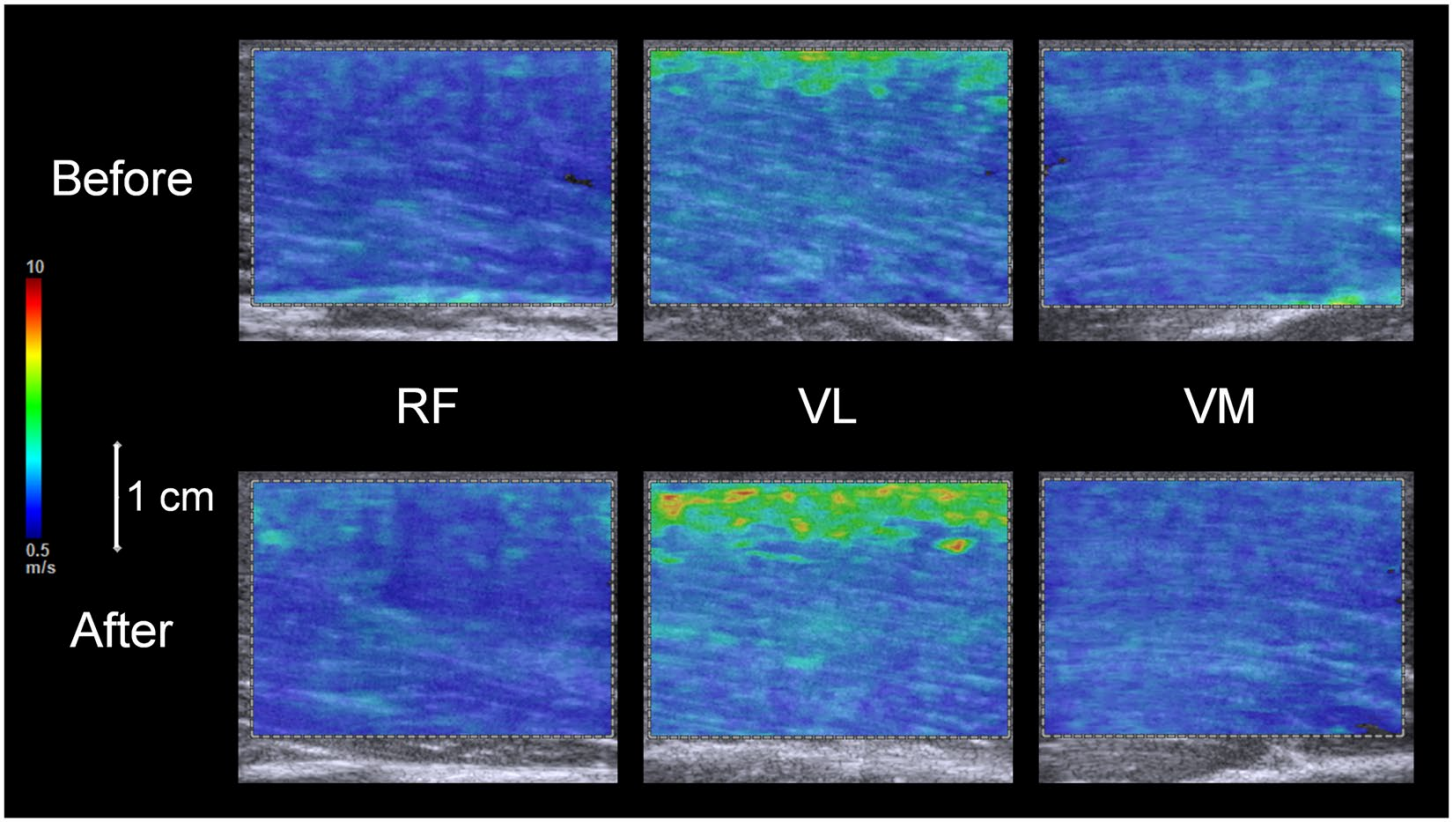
Typical elastography images of the rectus femoris and lateral and medial vastus muscles (RF, VL, and VM) before (upper) and after (lower) the fatiguing task. Shear wave ultrasound elastography generated color-coded images with a scale from blue (soft) to red (hard) depending on the magnitude of the shear wave propagation speed.
